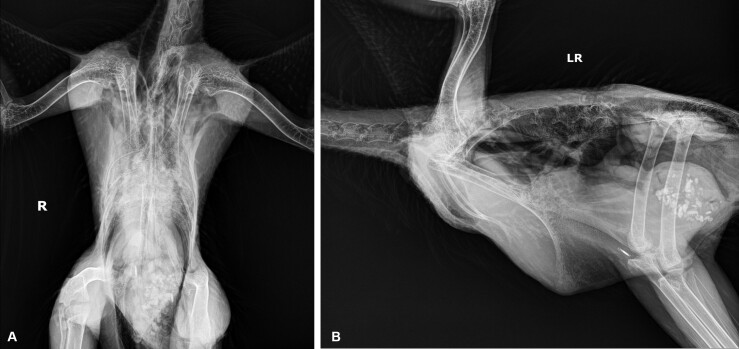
Radiographic findings in Curassow 2*.
